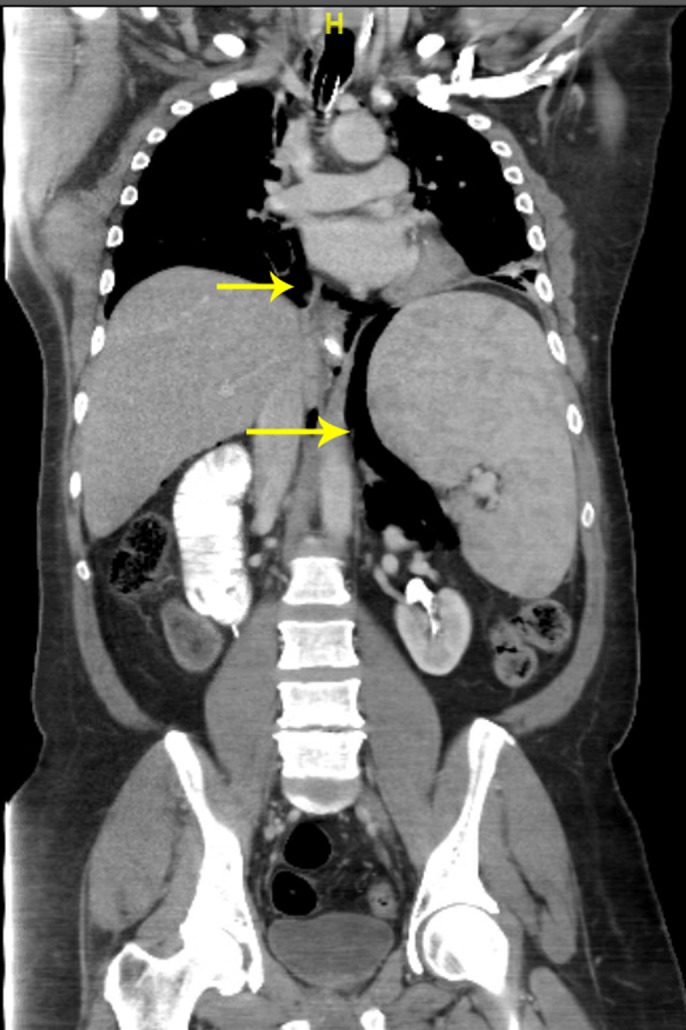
CT showing pneumomediastinum (top arrow), pneumoretroperitoneum (bottom arrow), following cryoablation. CT, computed tomography.
